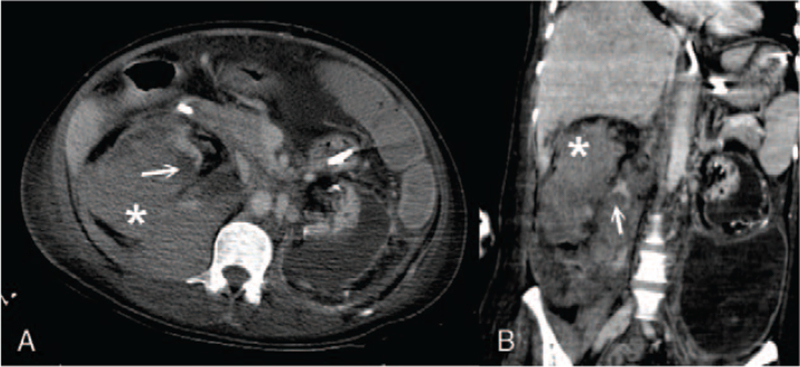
(A) Contrast extravasation of the right renal artery in computed tomography (transverse section). Arrow: Contrast extravasation of the right renal artery. Asterisk: Massive right renal hematoma. (B) Contrast extravasation of the right renal artery with massive right renal hematoma in computed tomography (coronal section). Arrow: Contrast extravasation of the right renal artery. Asterisk: Massive right renal hematoma.
